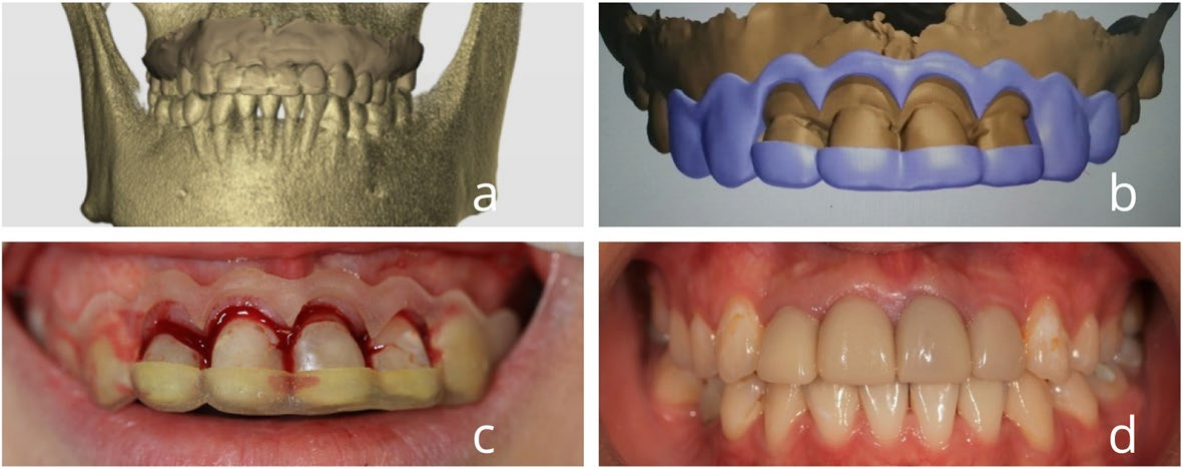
Dual positioning guide design and performing surgery. a-b Fitting CBCT and oral scan data and design (c) Crown lengthening surgery guided by digital dual positioning guides (c) Gingival contouring by wearing a 3D printed temporary crown
